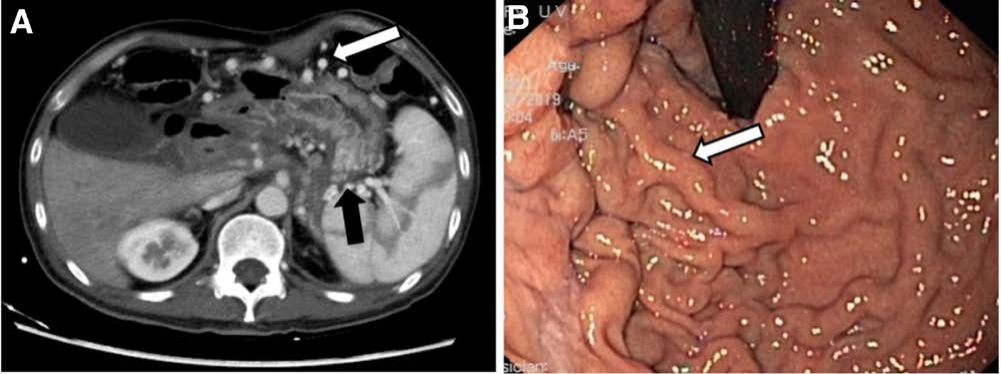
Abdominal enhanced CT and endoscopic images prior to splenic venoplasty. (A) Axial contrast enhanced CT abdomen at the level of renal hilum venous stage showed tortuosity and dilatation of fundus gastric veins (black arrow) and gastroepiploic veins (white arrow), and unclear splenic veins. (B) Endoscopic images showing severe varicose gastric fundus veins (white arrow).
